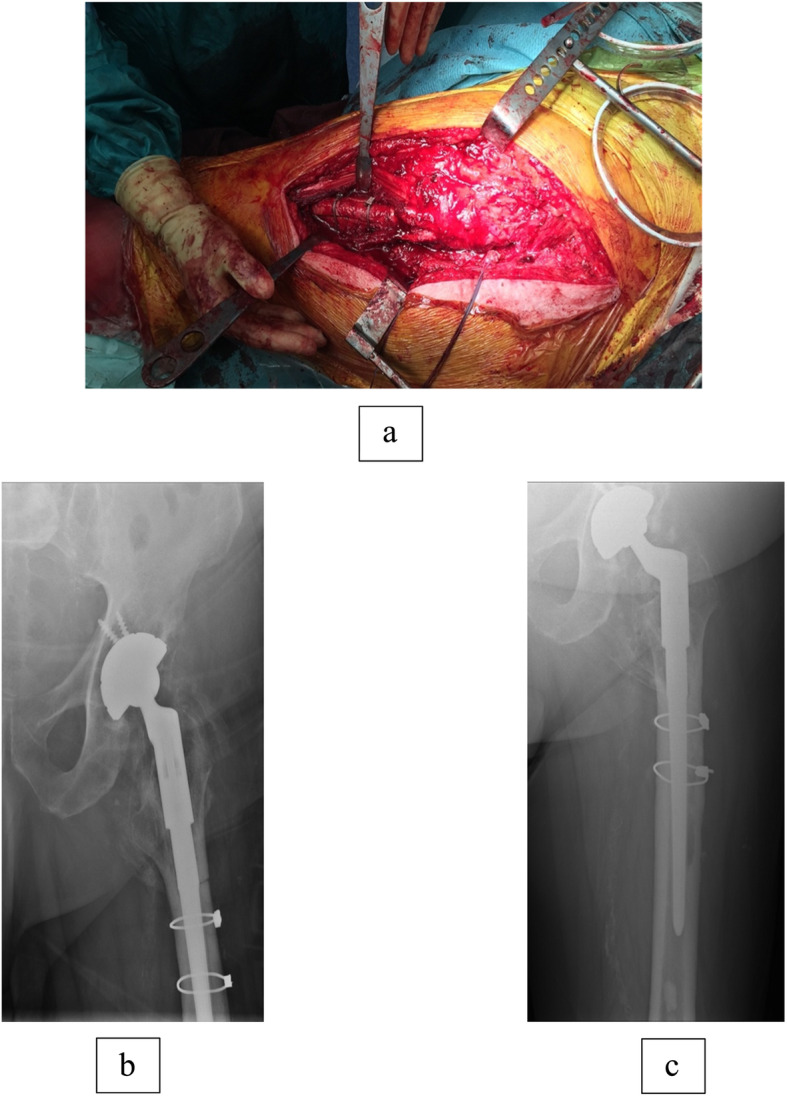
a Intraoperative image of stabilization of window with cerclage wires. b Immediate post-operative radiographs. c Two years follow-up radiograph with healed cortical window osteotomy
